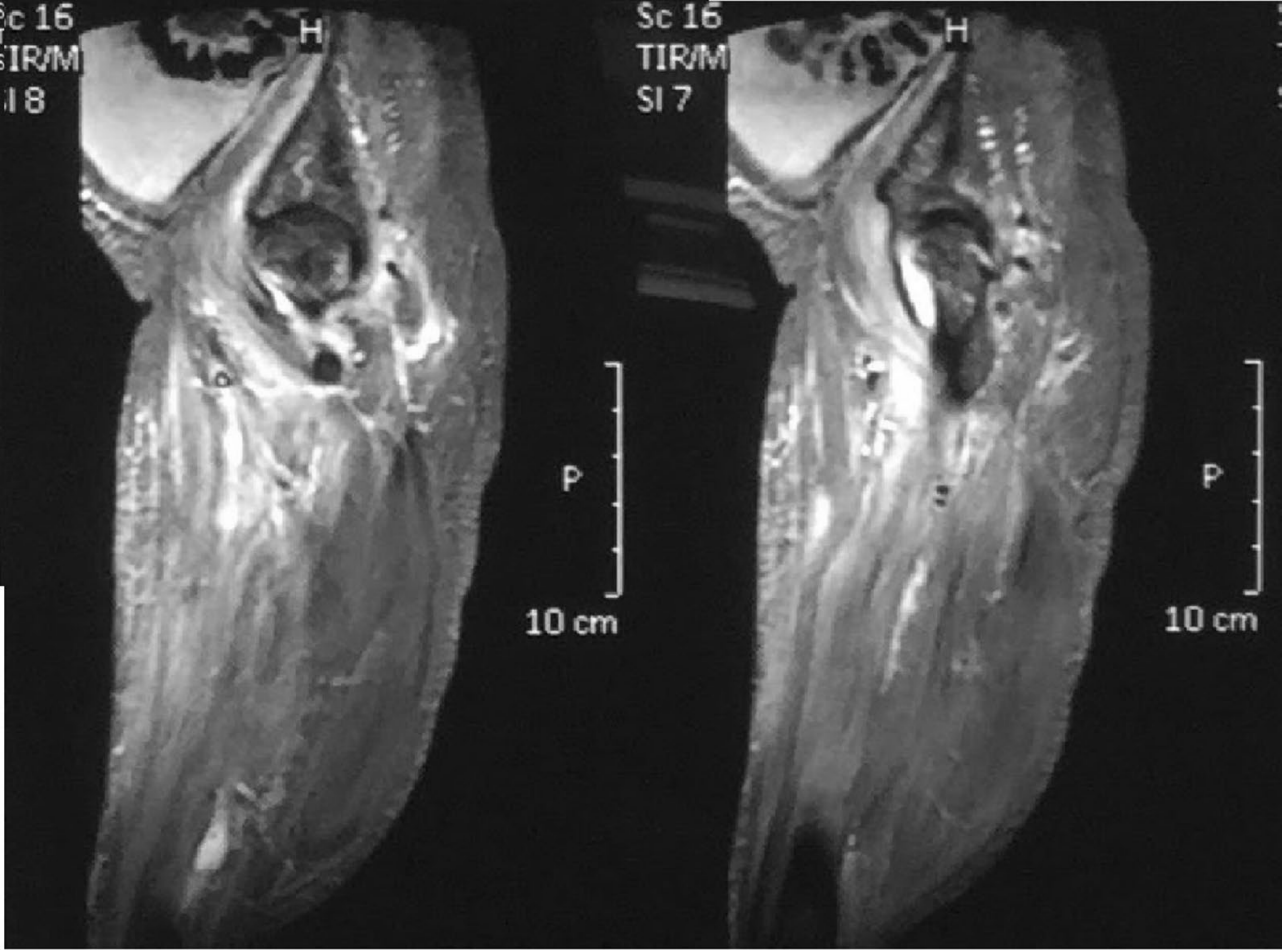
A coronal enhanced T1-weighted MRI image shows severe erosive bone changes, diffuse joint space narrowing and a small amount of joint effusion with synovial enhancement
